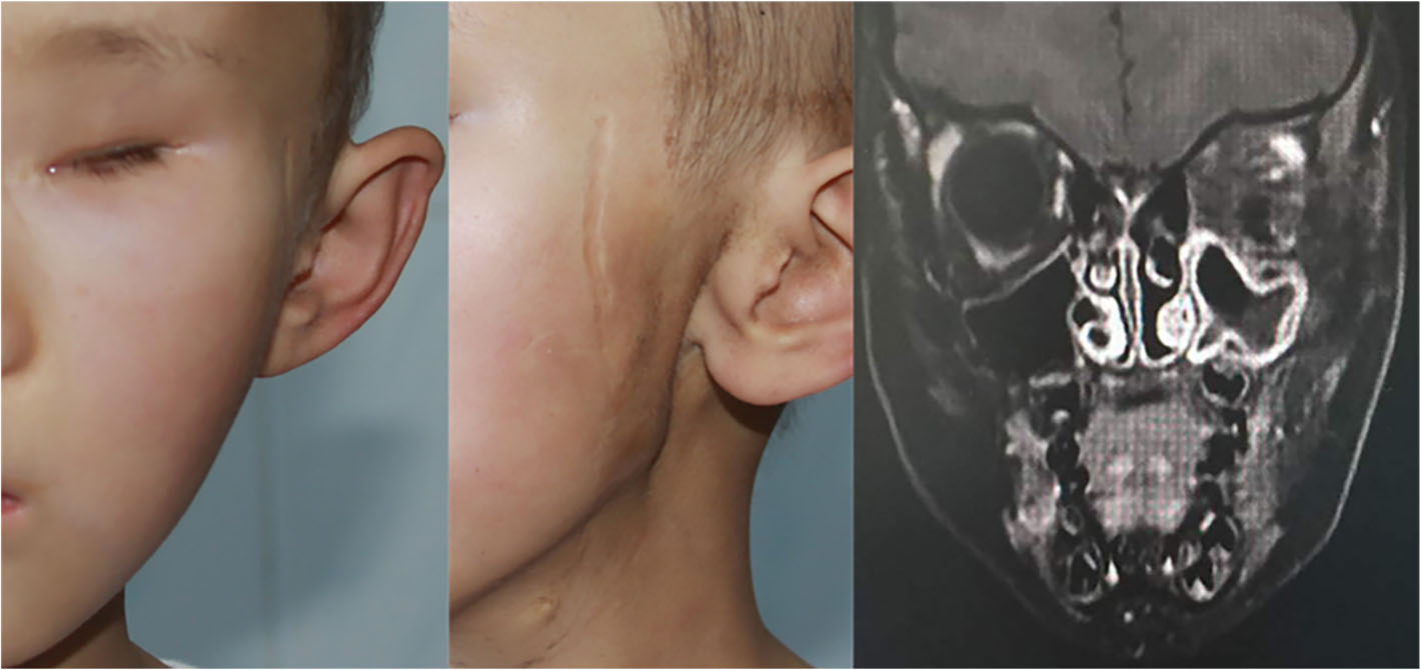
(April 2017) Clinical photograph of the girl at 28 months after operation. MRI scan shows no clear change compared with Fig. 3
